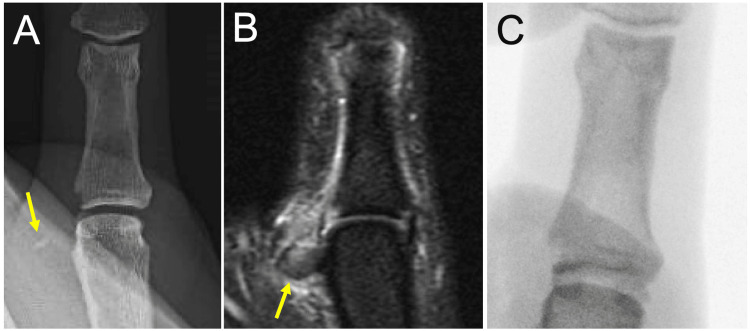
X-rays (A) demonstrating bony UCL avulsion (yellow arrow), with Stener lesion on MRI (B, yellow arrow). Intraoperative fluoroscopy image following repair (C). UCL, ulnar collateral ligament; MRI, magnetic resonance imaging.
